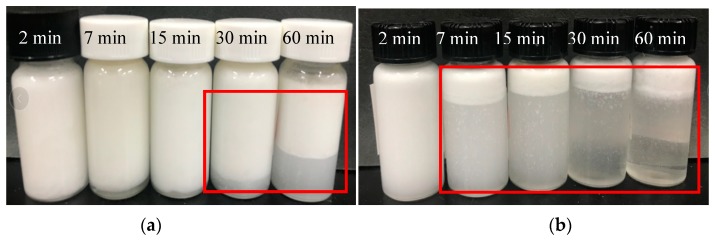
General appearance of 5% coconut oil O/W emulsions prepared by different ultrasonic intensities and treatment times after 30 days of storage at 30 °C: (a) 270 W and (b) 390 W.
